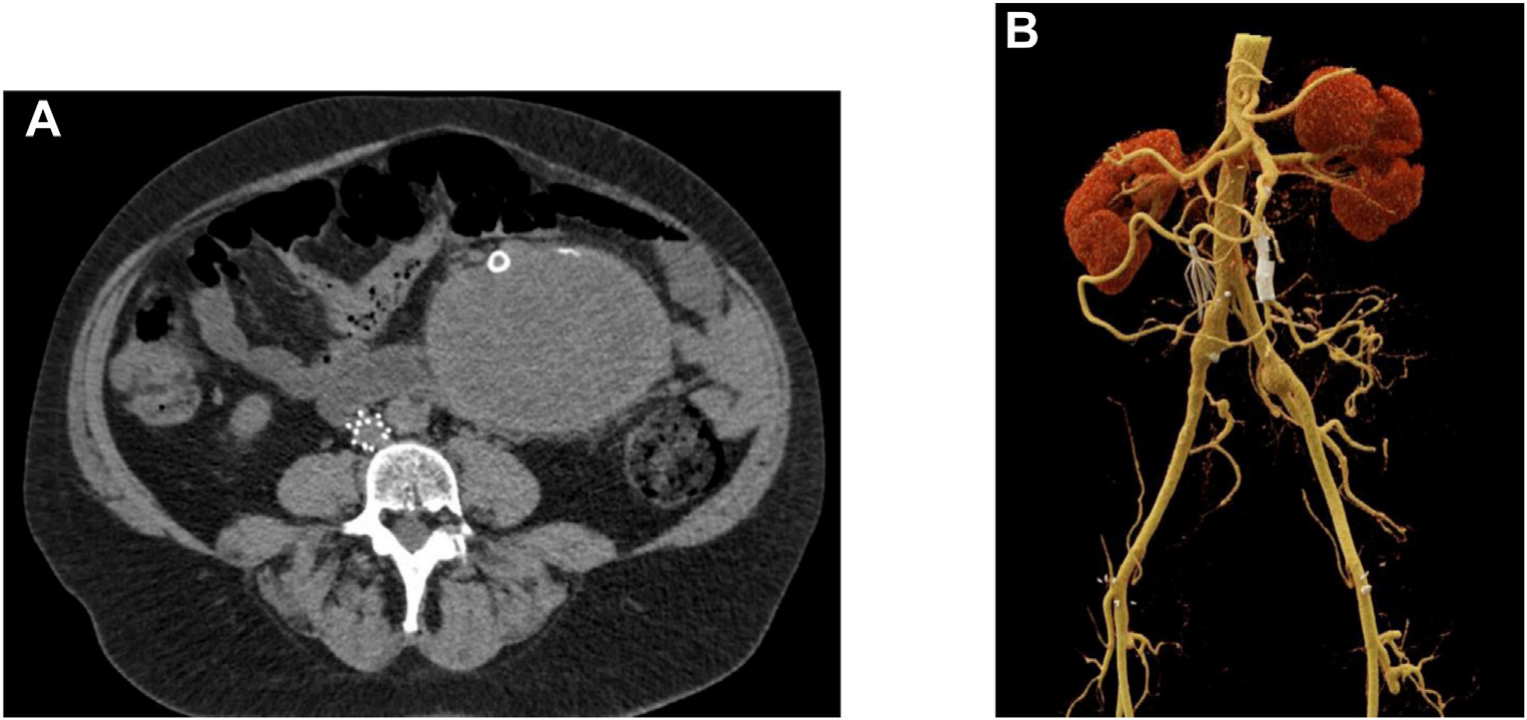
A and B, Axial view of follow-up abdominal computed tomography angiography (CTA) with aneurysm sac regression and superior mesenteric artery (SMA) stent patency. Three-dimensional postoperative reconstruction of SMA endovascular repair.
